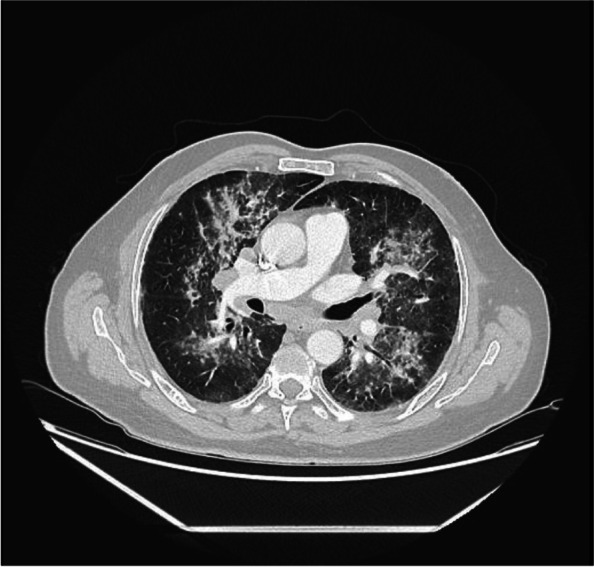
CT pulmonary angiography at presentation showing diffuse patchy consolidation with few reactive lymph nodes
